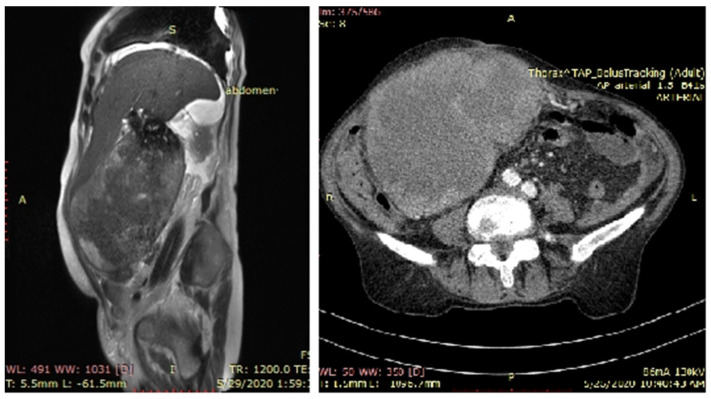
MRI (left) and CT (right) images, sagittal section and cross-section. A large subhepatic tumor mass occupied the entire flank and right hypochondrium, with the blurring of the demarcation line from the colon, duodenum, and jejunum, which appeared invaded by the tumor mass.
